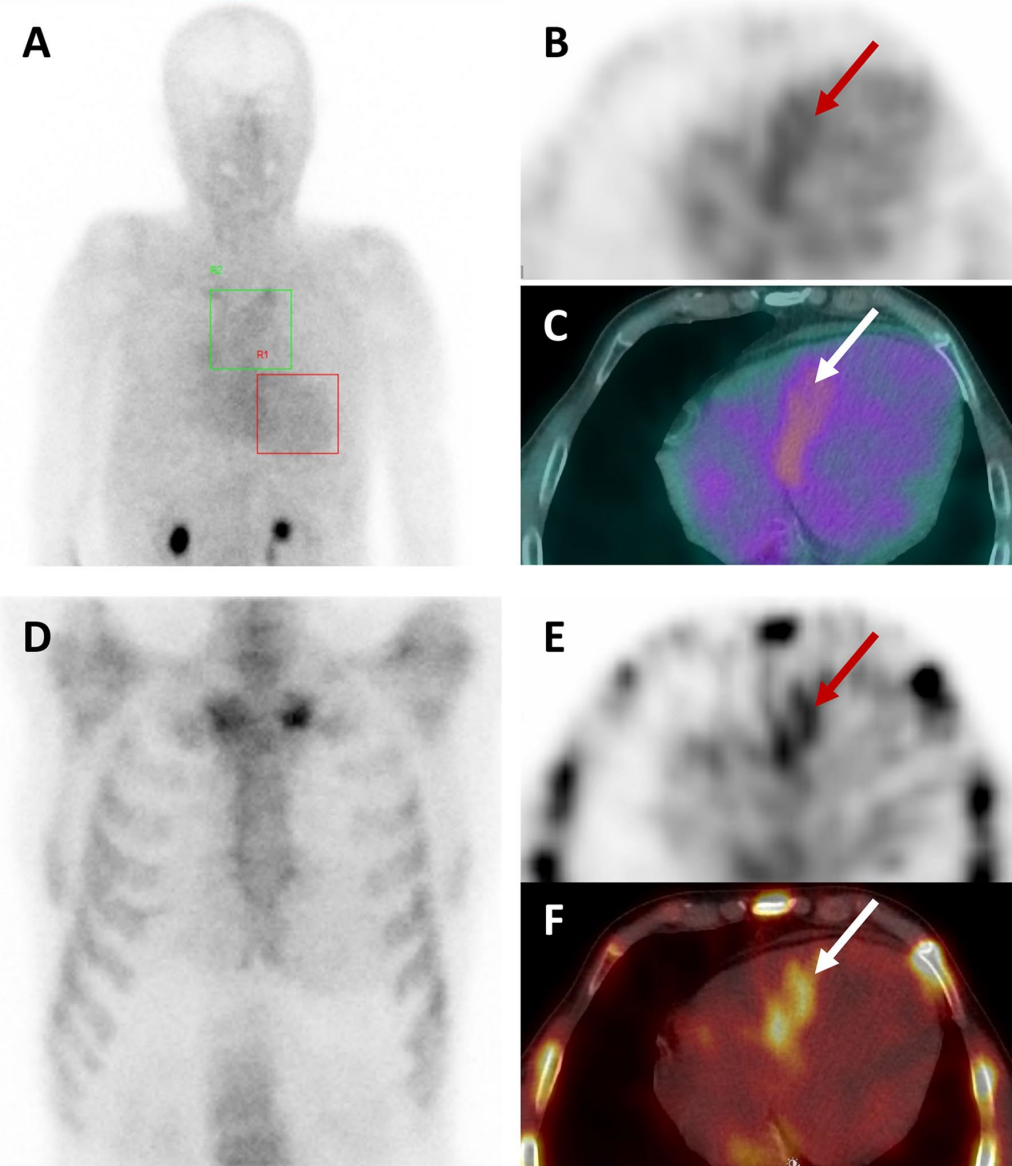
An example of false negative bone scintigraphy (patient #12). A Early ^99m^TC-HMDP-BS planar imaging with no cardiac uptake and an H/M ratio of 1.02. B, C Early ^99m^TC-HMDP-BS SPECT imaging (B) and coupled CT (C), showing basal septal uptake (red and white arrows). D Late ^99m^TC-HMDP-BS planar imaging with no cardiac uptake E, F Late ^99m^TC-HMDP-BS SPECT imaging (E) coupled with CT (F), showing the same basal septal uptake (red and white arrows)
